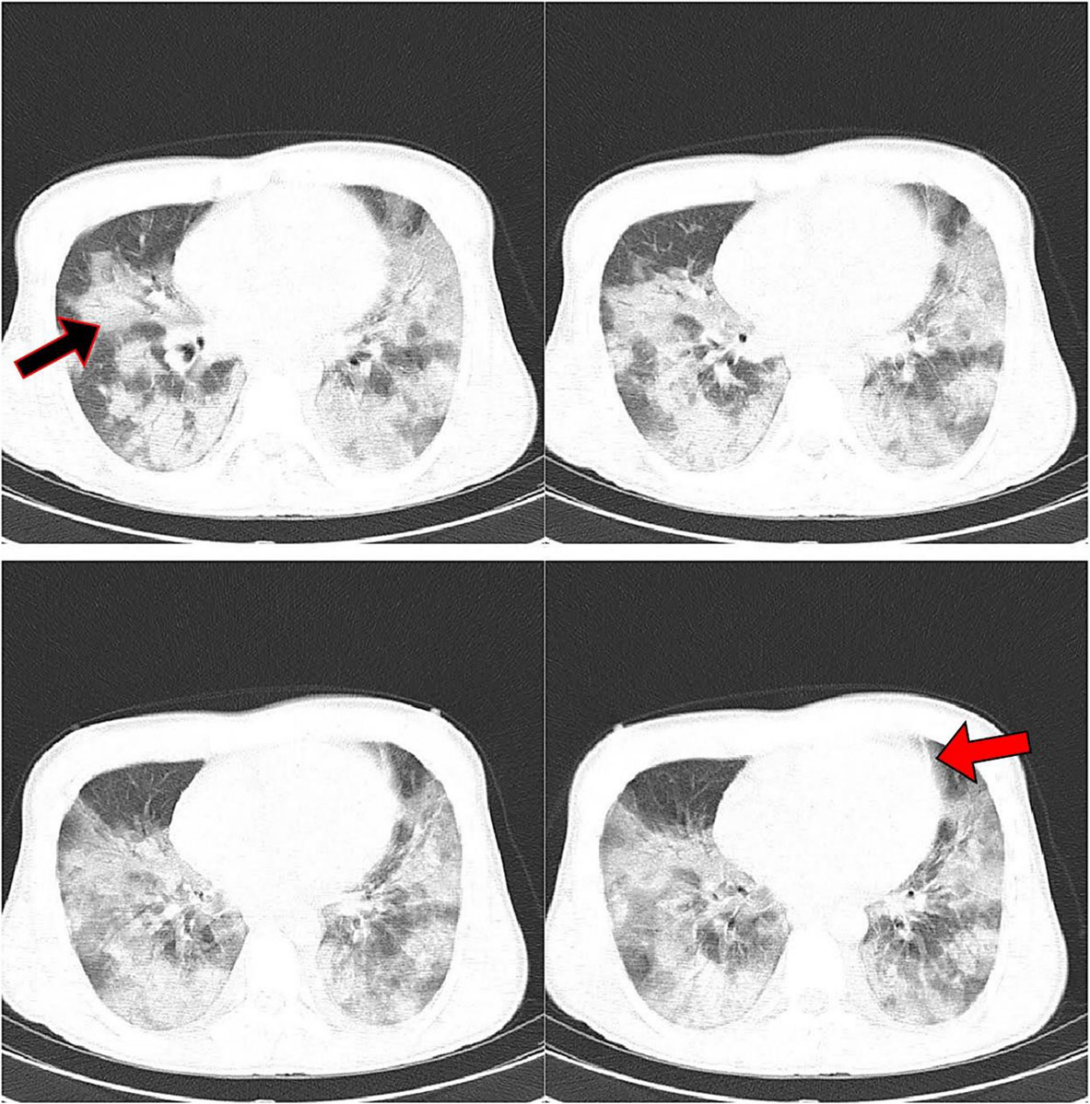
A 34-year-old male patient presented with fever, chills, myalgia, and dyspnea, who was admitted to ICU for 6 days and was discharged on day 21 of admission. Peripheral and peribronchovascular multilobar ground glass opacities (black arrow), and parenchymal bands (red arrow) are observed in the CT scan. The total CT severity score was calculated as 23, and the disease severity was severe
